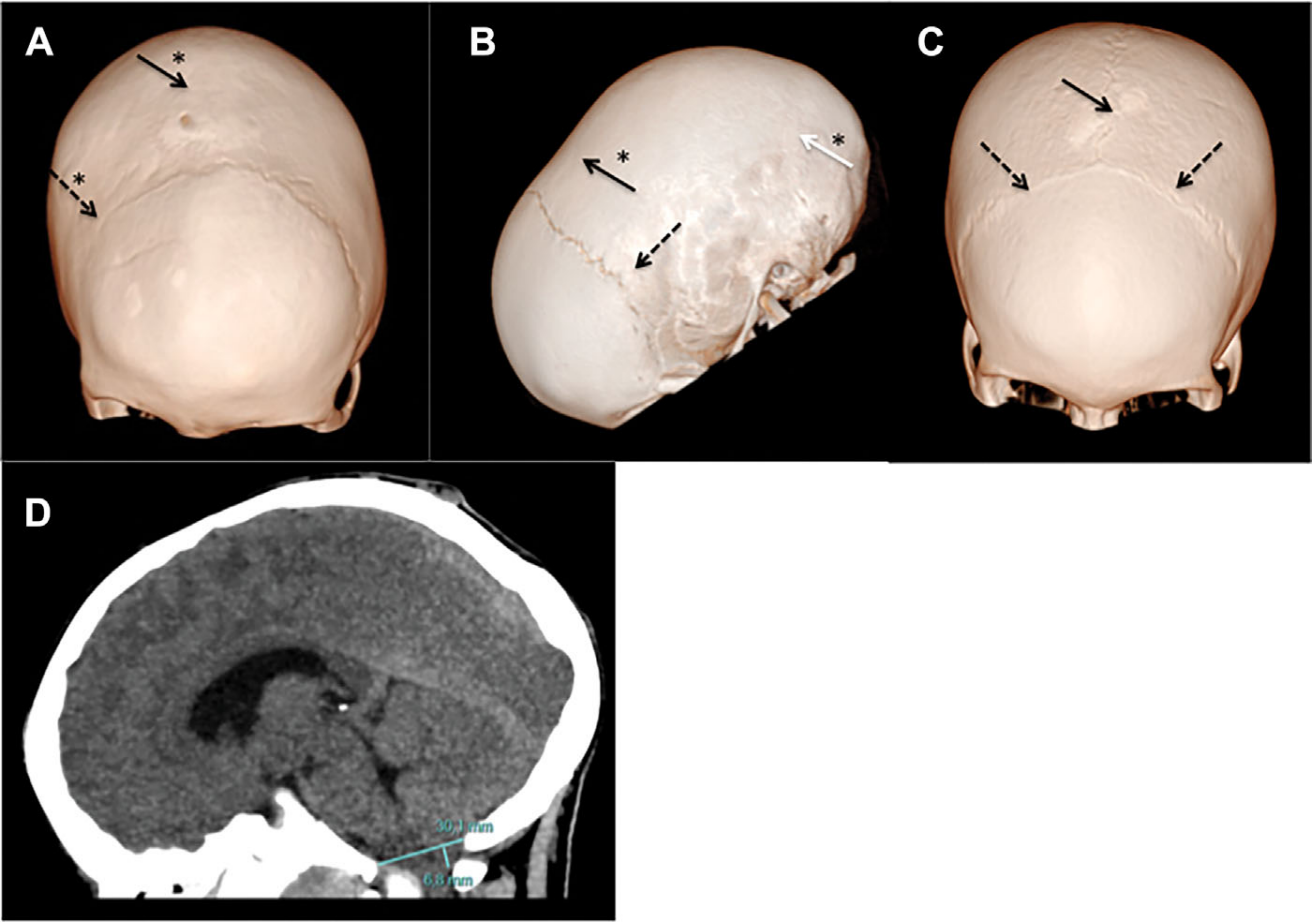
(*A*) An 8‐year‐old XLHR patient. Three‐dimensional CT skull bone window reconstruction. Complete closure of the sagittal and the right coronal sutures. The left coronal suture is patent but narrow. The patient also shows a congenital parietal foramina. (*B*) A 6‐year‐old XLHR patient. Three‐dimensional CT skull bone window reconstruction. Complete closure of the sagittal suture and both lambdoid sutures. (*C*) A 12‐year‐old XLHR patient. Three‐dimensional CT skull bone window reconstruction. Patent sagittal and coronal sutures. All very narrow. (*D*) An 8‐year‐old XLHR patient. Head CT sagittal slice parenchyma window showing a 6.8‐mm descent of the cerebellar tonsils. Continuous black arrows indicate sagittal suture. Discontinuous black arrows indicate coronal sutures. Continuous white arrow indicates lambdoid suture. Asterisks indicate closed sutures.
